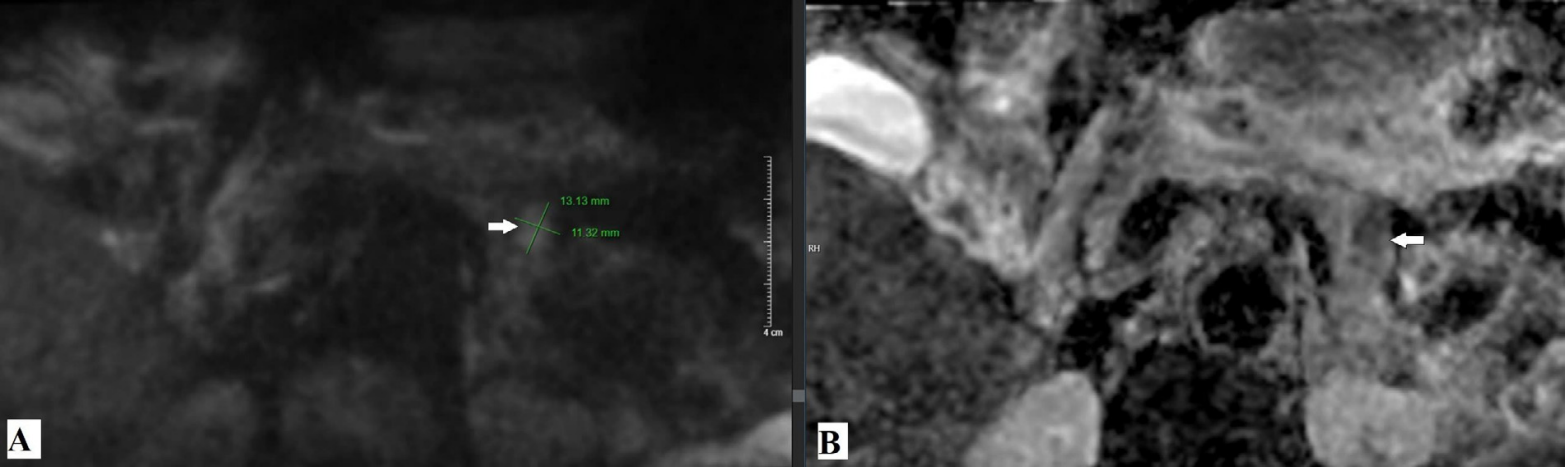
Small field-of-view (sFOV) diffusion-weighted imaging (DWI) sequence with a b-value of 1000 s/mm².(A) The image demonstrates a focal area of restricted diffusion located between the pancreatic body and tail, measuring approximately 13 × 11 mm (white arrow). (B) The corresponding apparent diffusion coefficient (ADC) map shows a hypointense region in the same location (white arrow).
